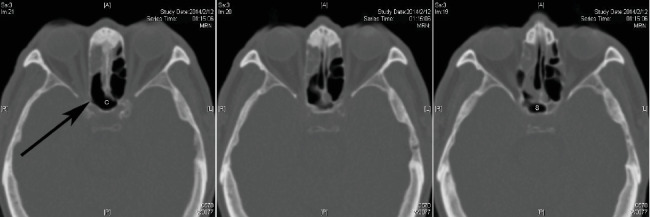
Three consecutive up-down CT images of an example of central sphenoethmoidal cell (C) that lies superiorly, extends more than 5 mm to the sphenoid sinus (S), and contacts right optic canal (arrow).
